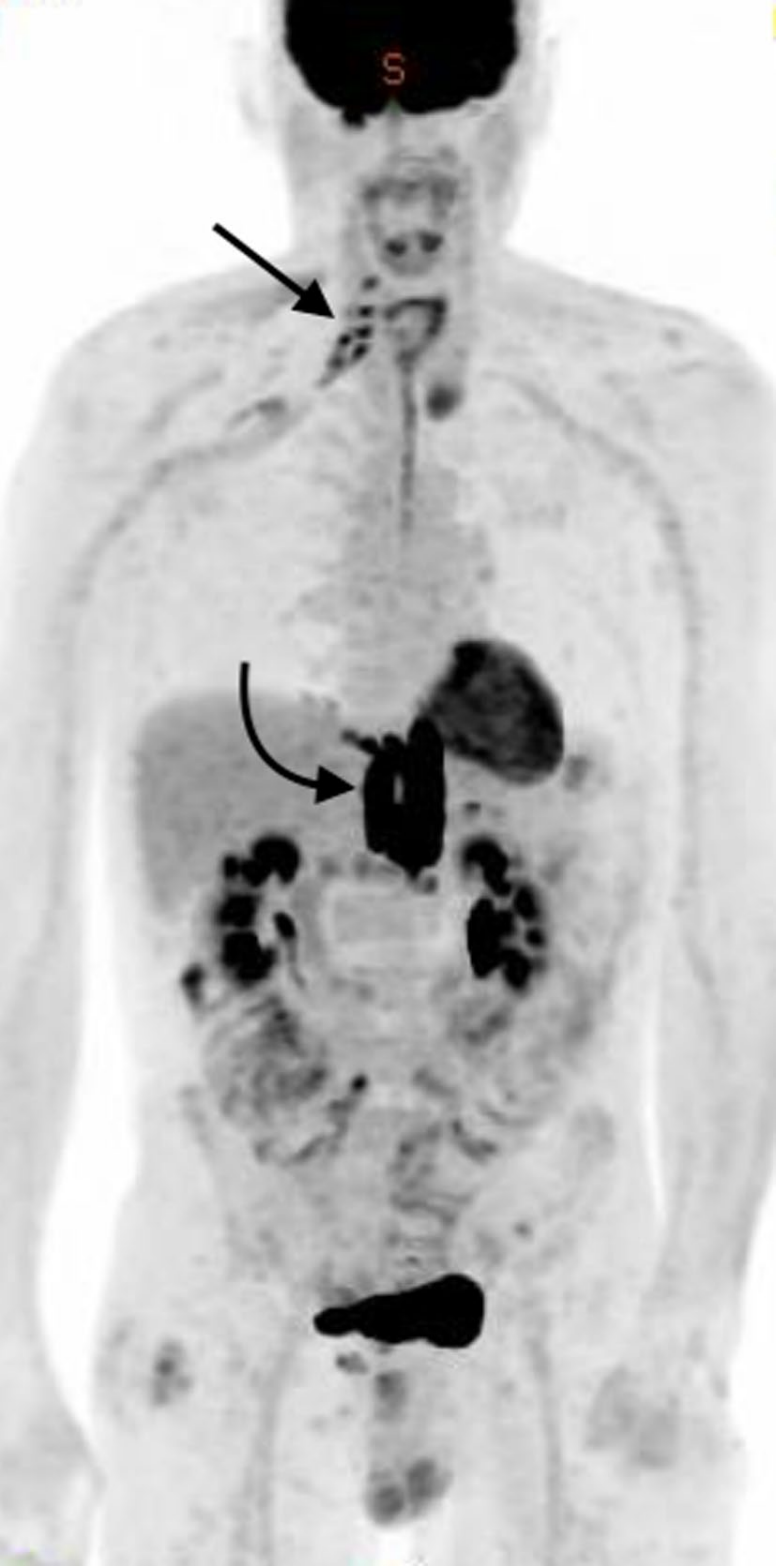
MIP image from whole-body ^18^F-FDG-PET/CT demonstrates claw-shaped asymmetrical right sided, multi-segmental intense linear ^18^F-FDG uptake, in the distribution of C5 to T1 nerve roots of the brachial plexus (straight arrow) and nodal recurrence below the diaphragm (curved arrow)
